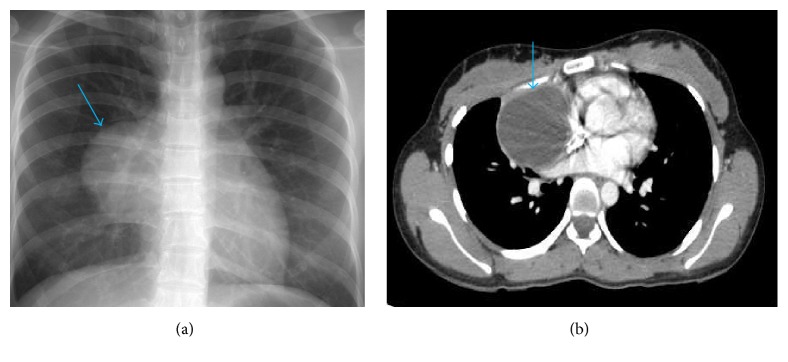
(a) Chest X-ray showed 6 × 4.5 cm white opaque lesion in mediastinal area (blue arrow) and (b) CT-scan revealed hypodense mass 6.7 × 7.5 cm, suggesting inferoanterior mediastinal cyst (blue arrow). Encapsulated cystic lesion compressed adjacent aorta and pericardium.
